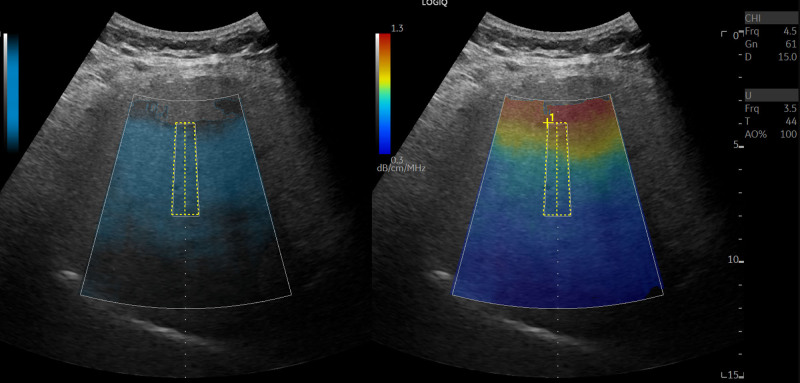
UGAP measurements. A large color-coded attenuation map was positioned for UGAP measurement in a homogeneous area of the liver, free of large vessels. UGAP = ultrasound-guided attenuation parameter.
